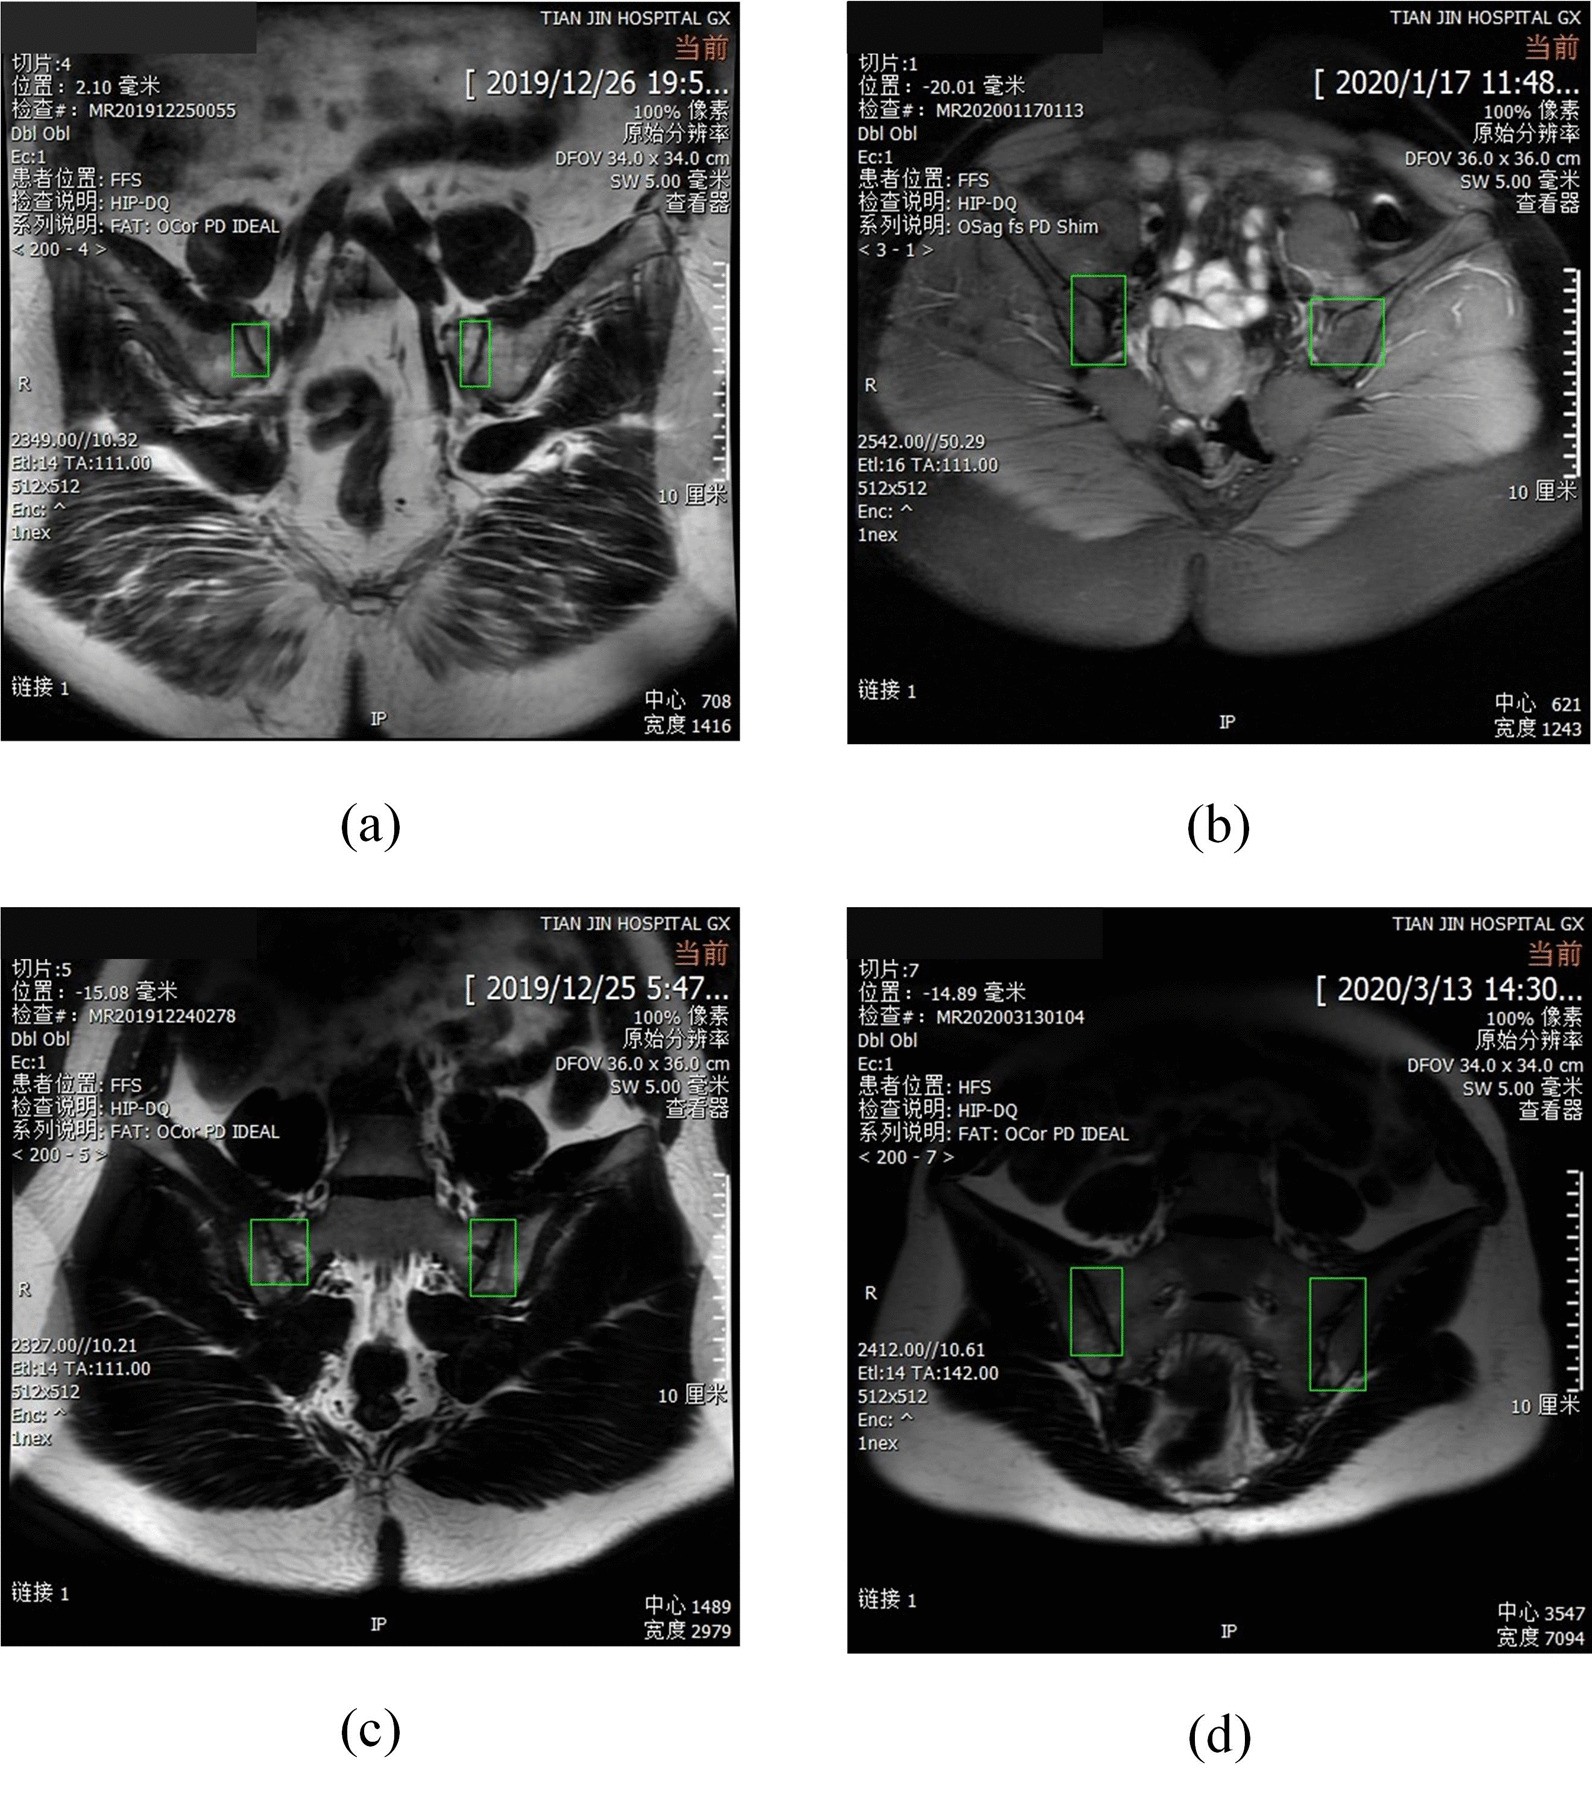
Different styles of MRI images and different shapes of bone marrow edema. a Easily distinguishable bone marrow oedema; b Indistinguishable bone marrow oedema; c Indistinguishable bone marrow oedema; d Indistinguishable bone marrow oedema
